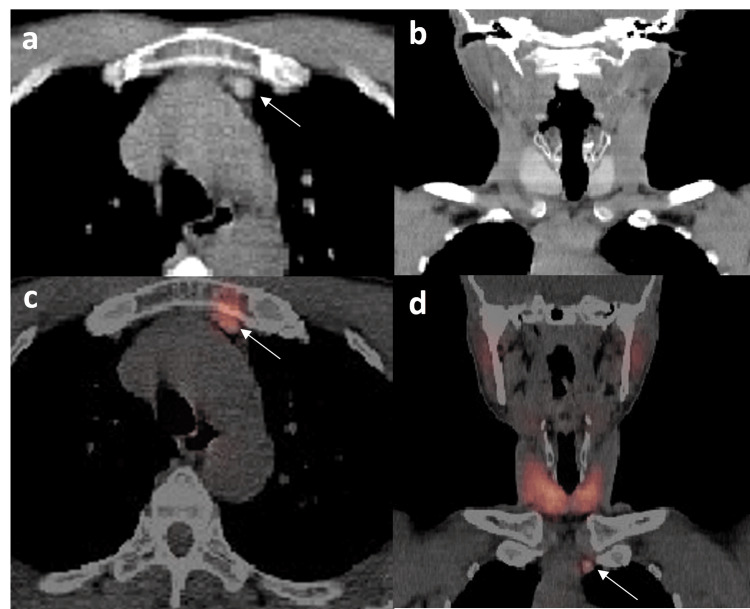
Axial CT (a), coronal CT (b), axial fused SPECT/CT (c), and coronal fused SPECT/CT, and (d) reconstruction of SPECT/CT scan of the neck and upper chest. Soft tissue nodule (white arrow) in the pre-vascular space with similar density and uptake to the thyroid gland.
